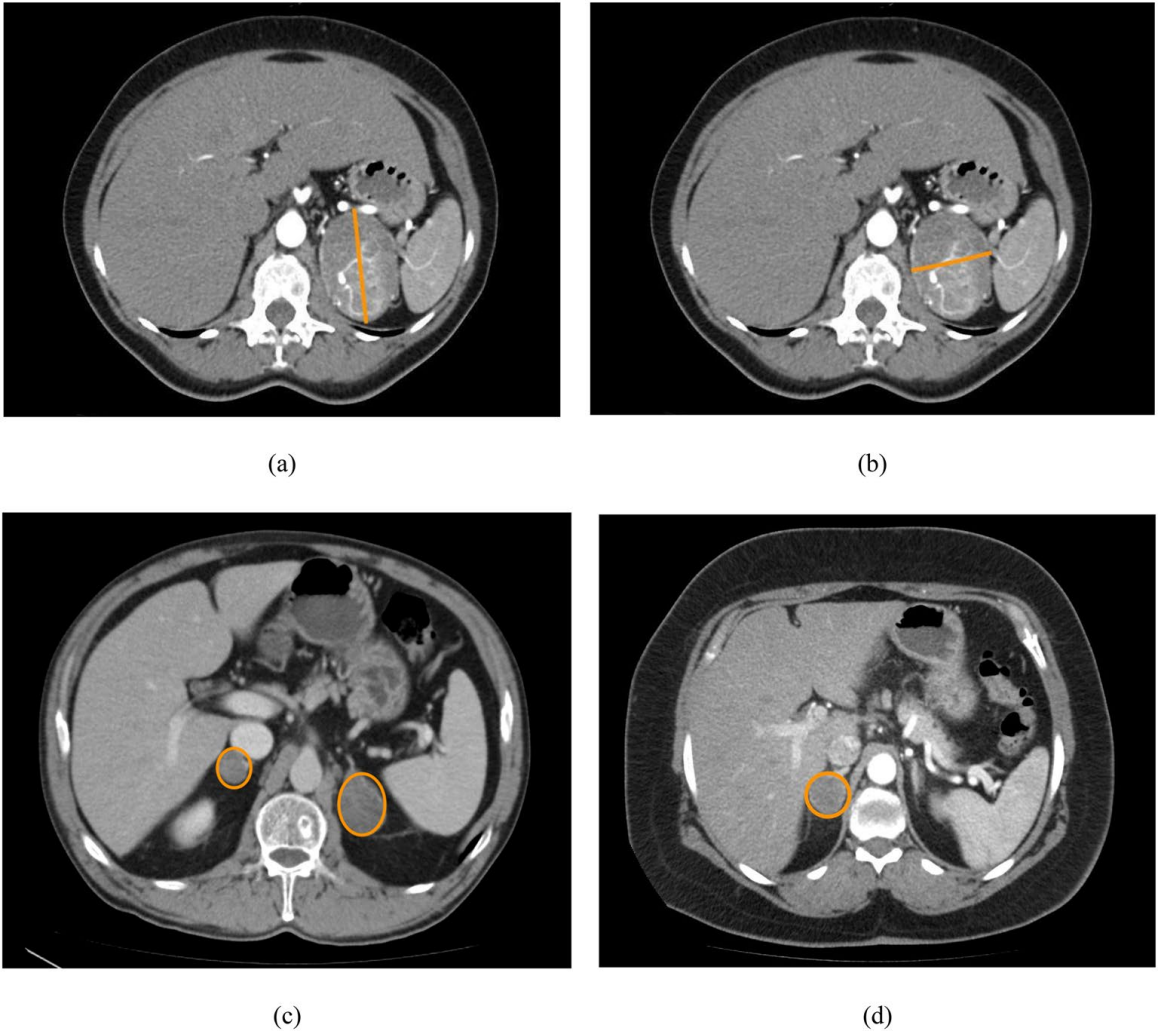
Examples CT image with adrenal tumour showing attributes used in this study: (a) maximal diameter for tumour with homogeneity feature absent and laterization feature present, (b) minimal diameter for tumour with homogeneity feature absent and laterization feature present, (c) tumour with laterization feature absent, (d) tumour with homogeneity feature present.
